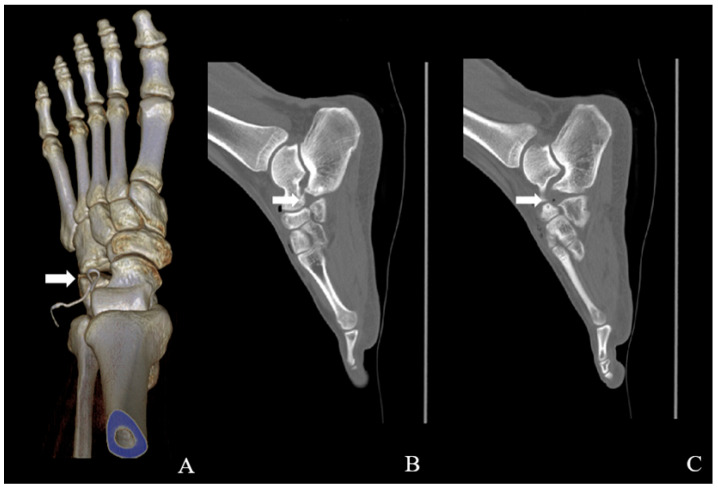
Postoperative computed tomography images of the left foot. (A) Three-dimensional reconstructed image demonstrates the calcaneus following surgical excision of the accessory ossicles (arrow). (B,C) Sagittal CT images show complete removal of the previously identified ossicles, with no residual ossicle or bony fragment at the anterior process of the calcaneus (arrow).
